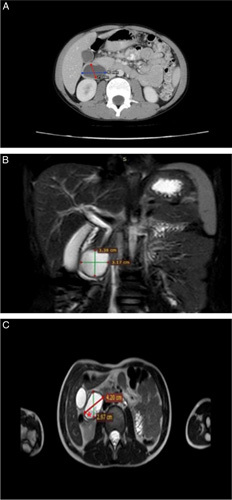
(A) Computed tomography scan with oral and IV contrast shows a 2.3 cm anteroposterior ×4 cm transverse well-defined cystic lesion posteroinferior to the pancreatic head. The cyst is intimately related to the second part of the duodenum but not contrasted with oral contrast. (B and C) MRCP shows a well-defined fluid-filled cystic structure between the head of the pancreas and the second/third duodenal junction measuring about 4.2 cm transverse ×3.38 cm craniocaudal ×2.97 cm anteroposterior in maximal dimensions. The cyst shows dependent debris without definite air bubbles (red asterisk) with a mass effect on the duodenum and head of the pancreas, resulting in minimal dilatation of the common bile duct.
